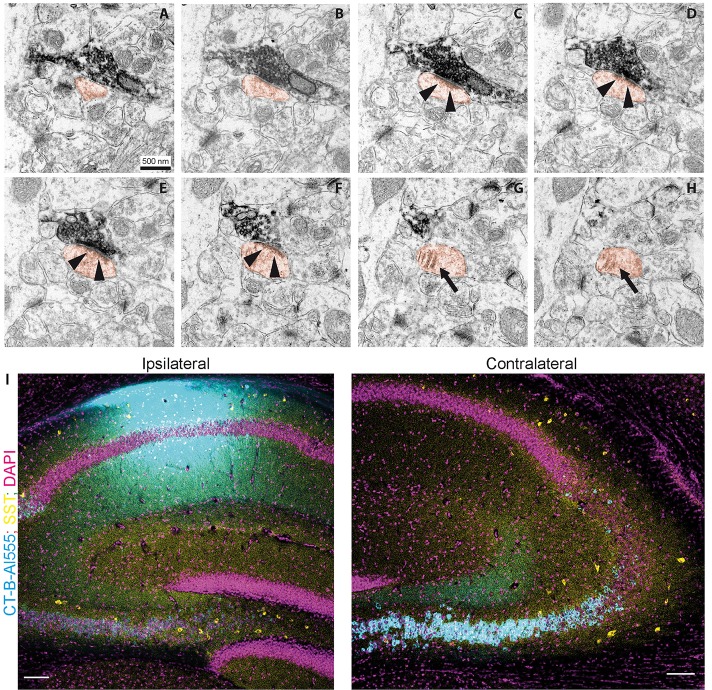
Contralaterally projecting axons originating from Cre-expressing neurons in CA3 are excitatory. (A–H) miniSOG positive electron-dense structure making presynaptic contact on a spine (orange) in CA1 SO. Arrows in (C–F) show postsynaptic density. Arrows in (G–H) show the spine apparatus. Scale bar: 500 nm. (I) Cholera toxin-B tracing in CA1. Ipsilateral injection of CT-B subunit in CA1. Contralateral, retrogradely traced cells (cyan) and SST immunoreactive cells (yellow). Scale bar: 200 μm.
